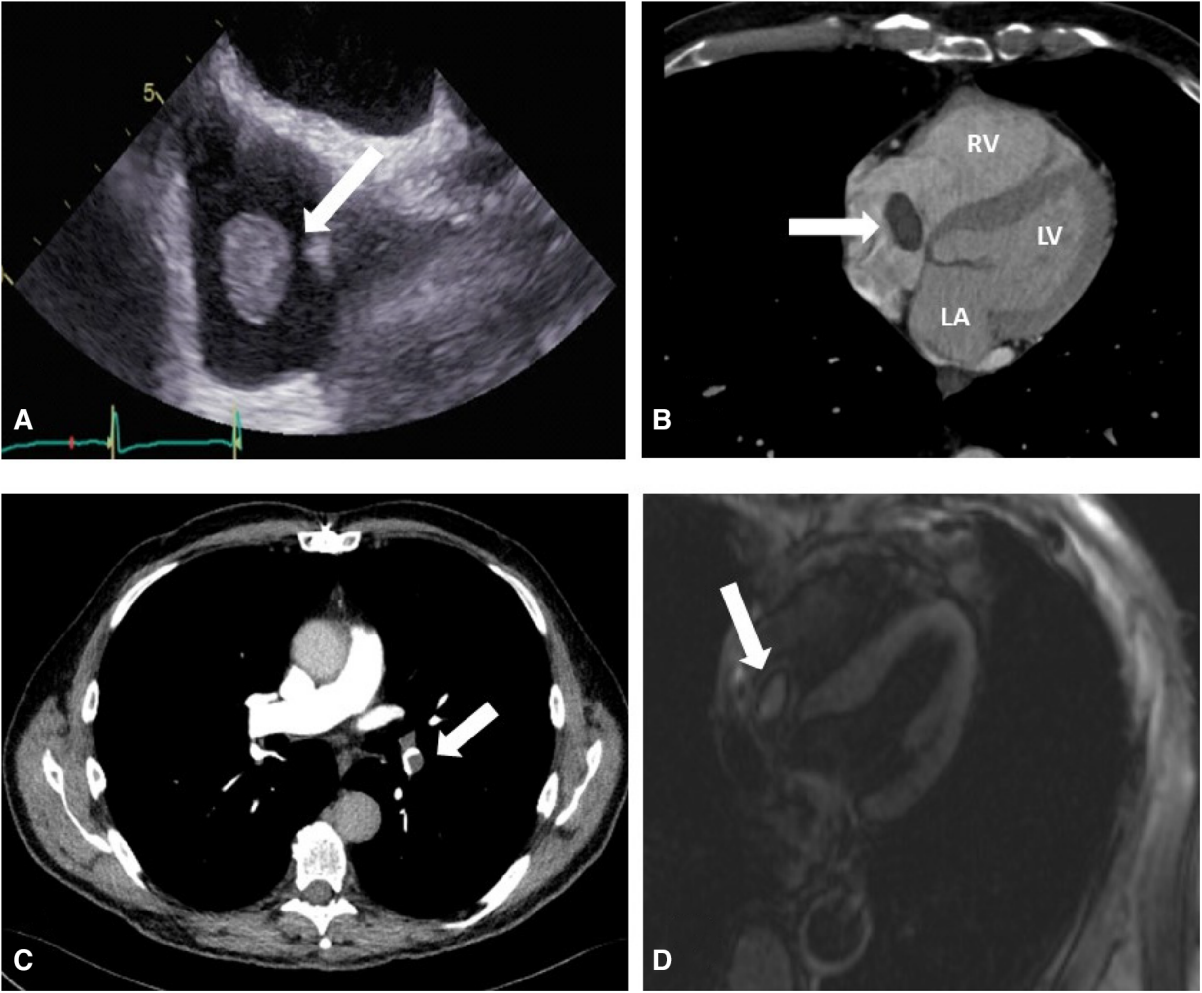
(A) Preoperative transesophageal echocardiography shows a mobile right atrial mass (arrow) on the Eustachian valve. (B) Preoperative thoracic CT angiography showing the right atrial mass (arrow), right ventricle (RV), left atrium (LA), and left ventricle (LV). (C) Preoperative thoracic CT angiography showing pulmonary embolization (PE) on the inferior branch of the left pulmonary artery (arrow). (D) MRI imaging that shows a central mass enhancement (arrow) on the Eustachian valve.
